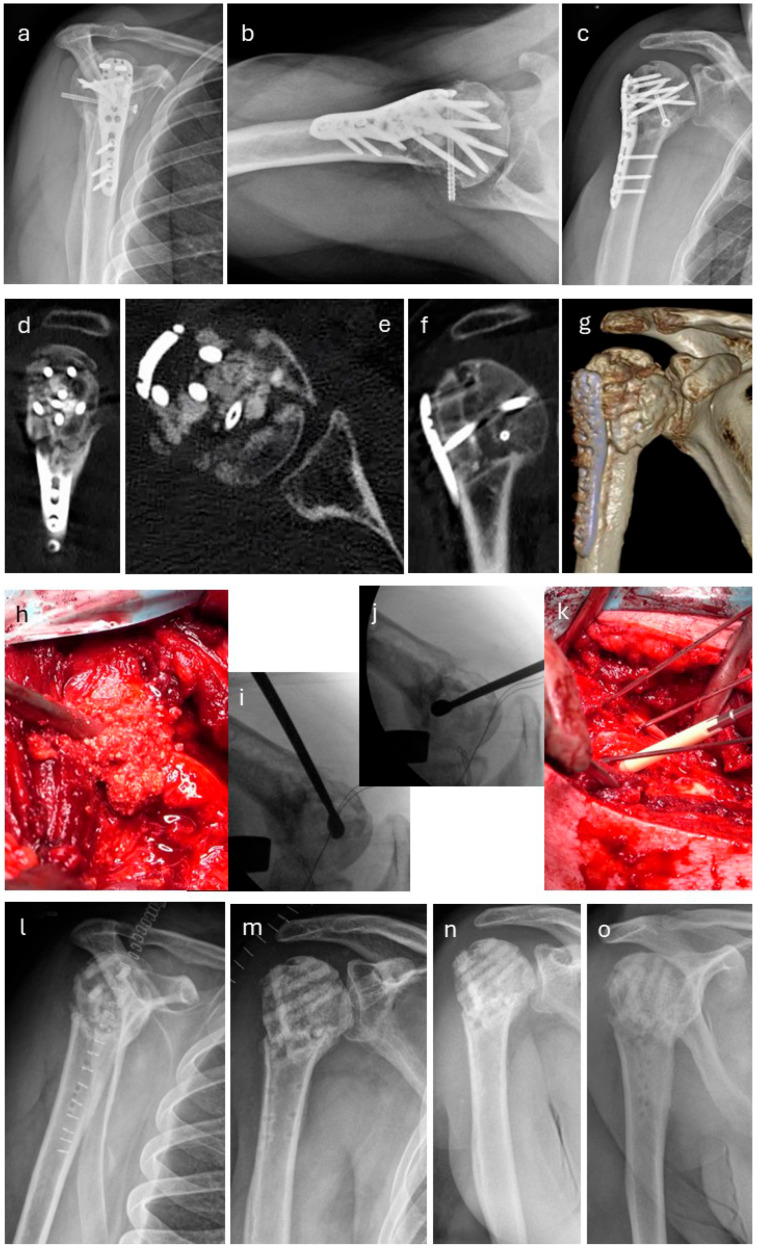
A case of a 44-year-old patient who had undergone surgery for a comminuted fracture of the right humerus, treated with a PHILOS^TM^ plate. (a–c): X-rays; (a): outlet view; (b): axial view; (c): anteroposterior view; the non-union failed to consolidate. (d–f): CT scans prior to revision; (d): sagittal view; (e): axial view; (f): coronal view; (g): CT 3D reconstruction; (h–k): eighteen months later, a single-stage procedure was performed, including removal of the bone substitute material (h), calcium phosphate, which, 18 months postoperatively, was non-vital, non-functional, and was not integrated into the patient’s bone metabolism, and hardware removal. (i,j): X-ray images during surgery demonstrate the extent of the defect cavity in the humeral head, depicted using a curette. (k): The pseudarthrosis is stabilized with five Kirschner wires, which are successively replaced by the transplantation of five human allogeneic cortical bone screws (Shark Screw^®^ DIVER) as cortical bone grafts for the bridging and fixation of the non-union, as well as the transplantation of human allogeneic bone granulate into the large humeral head defect. Follow-up radiographs (directly after surgery (l,m) and four weeks post-surgery (n,o)) after a fall onto the operated right shoulder show that the solely human biological osteosynthesis remains stable. The transplanted bone screws and the allogenic bone granulate have been partially remodelled by the host bone.
